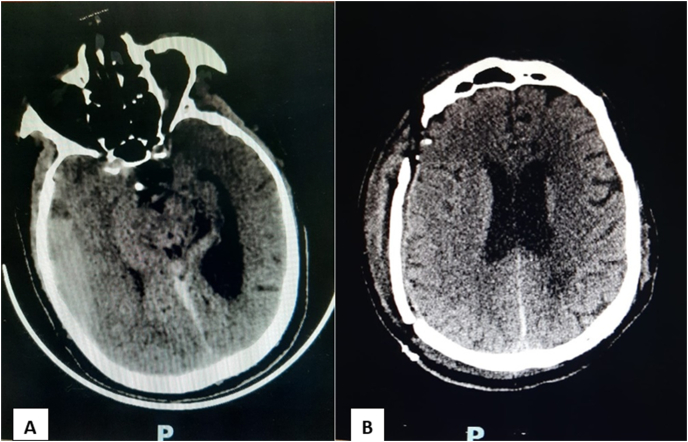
A) Preoperative brain computed tomography scan revealing a right temporoparietal acute subdural hematoma with mild mass effect on the ipsilateral ventricle. B) Postoperative brain computed tomography scan revealing complete resolution of subdural hematoma.
